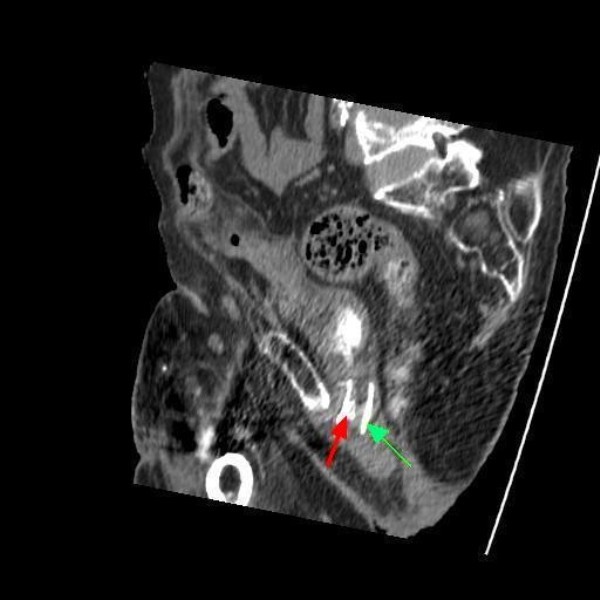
Computed tomography of pelvis (24 February 2009): sagittal section shows the stent in its long axis (green arrow) along with a wire protruding into the lumen of stent (red arrow).
